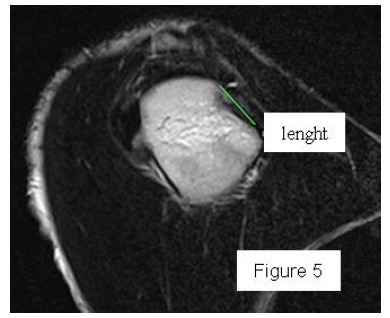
Measure of the lenght of Hill-Sachs lesion on CT sagittal view 55x46mm (150 x 150 DPI).
